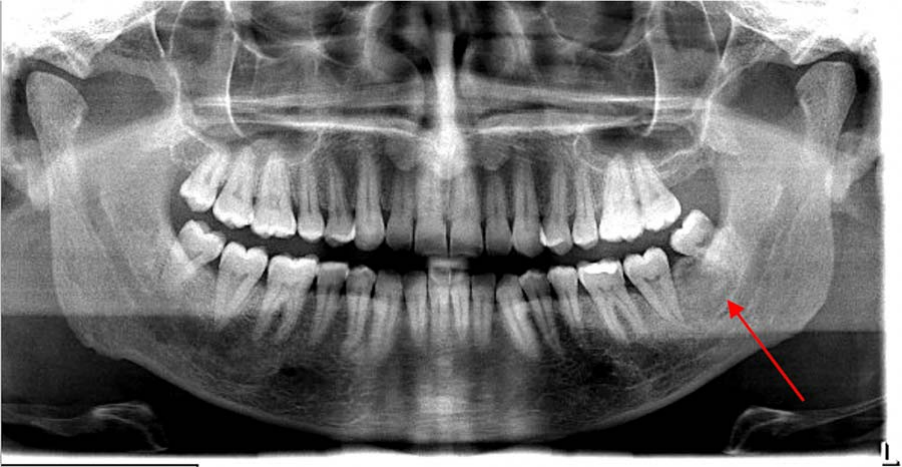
Orthopantomogram demonstrating an ill-defined lesion between the left lower molars (see arrow). This represents an ossifying fibroma.
